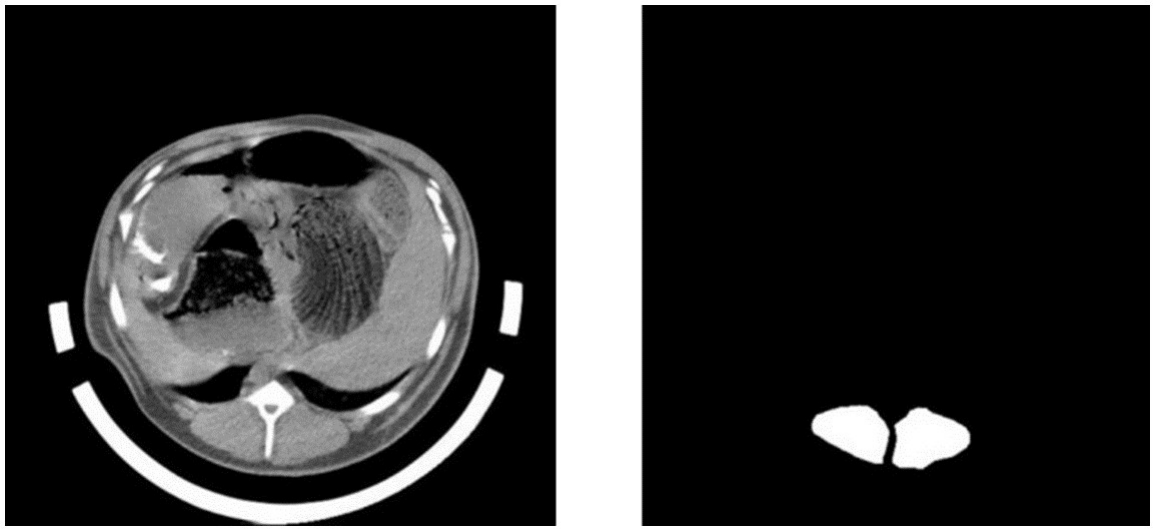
CT image and its annotated image.
